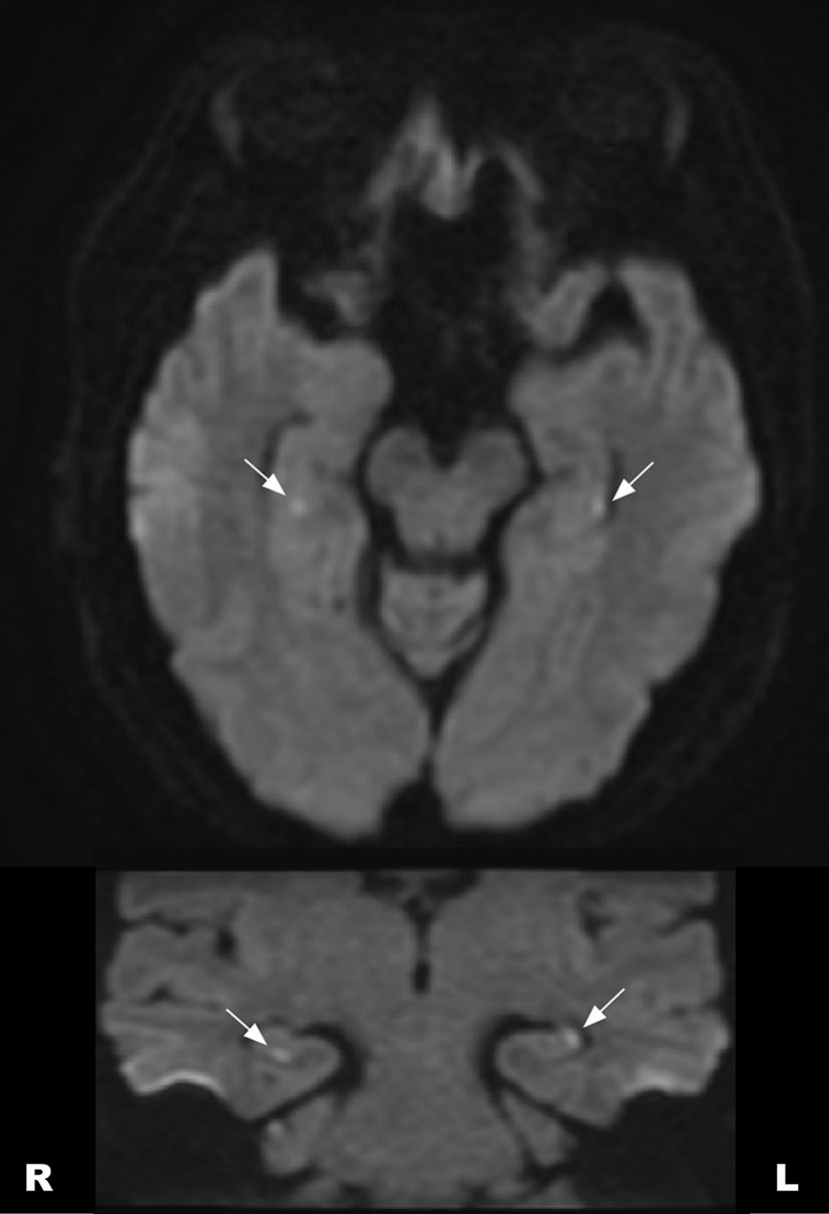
Representative diffusion-weighted MRI showing lesions in a TGA patient. Upper, axial slice; lower, coronal slice. *TGA* transient global amnesia, *MRI* magnetic resonance imaging. Arrows indicate hippocampal CA1 lesions.
